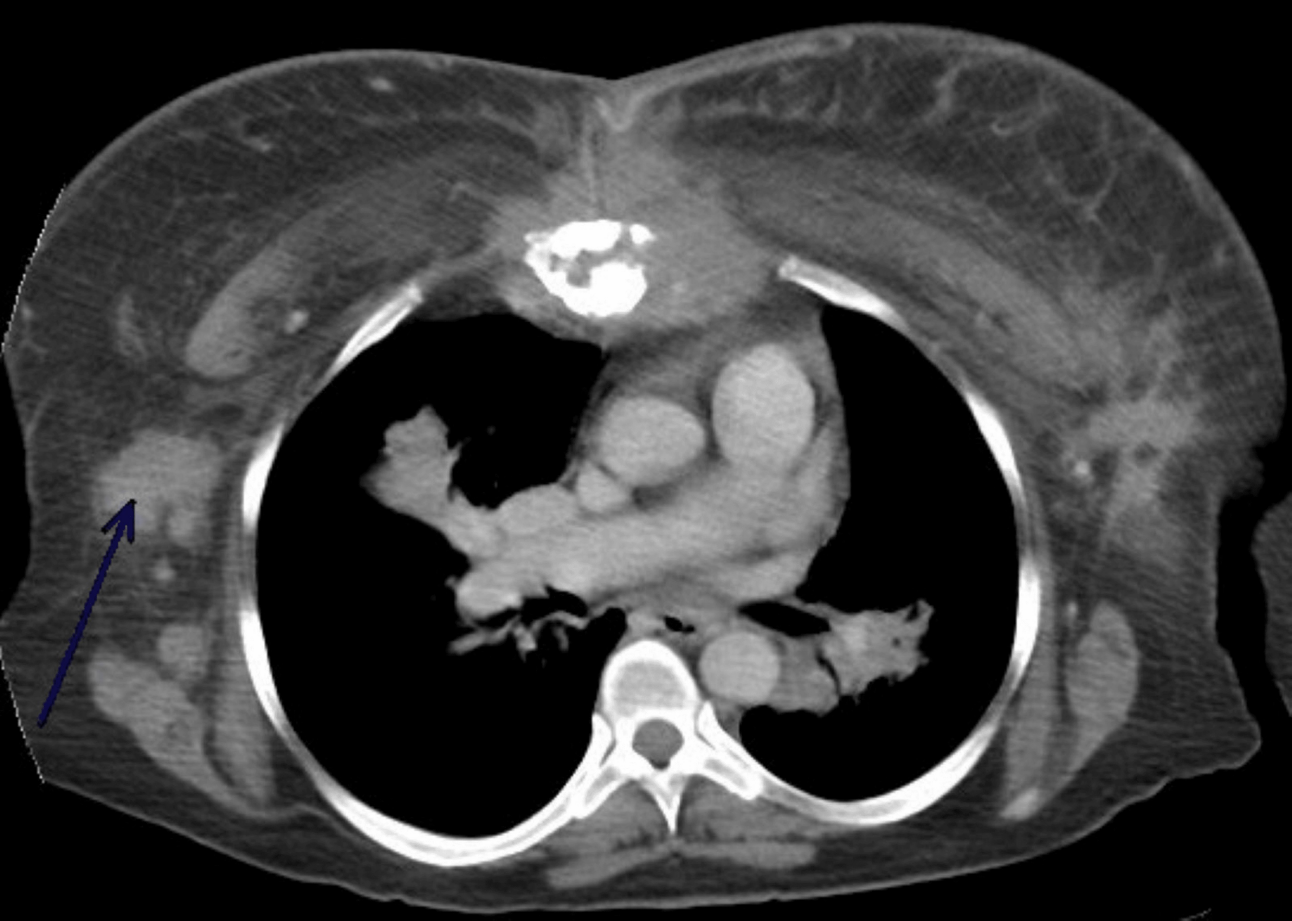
Computer tomography (CT) of the chest shows bilateral breast masses consistent with possible breast cancer.
